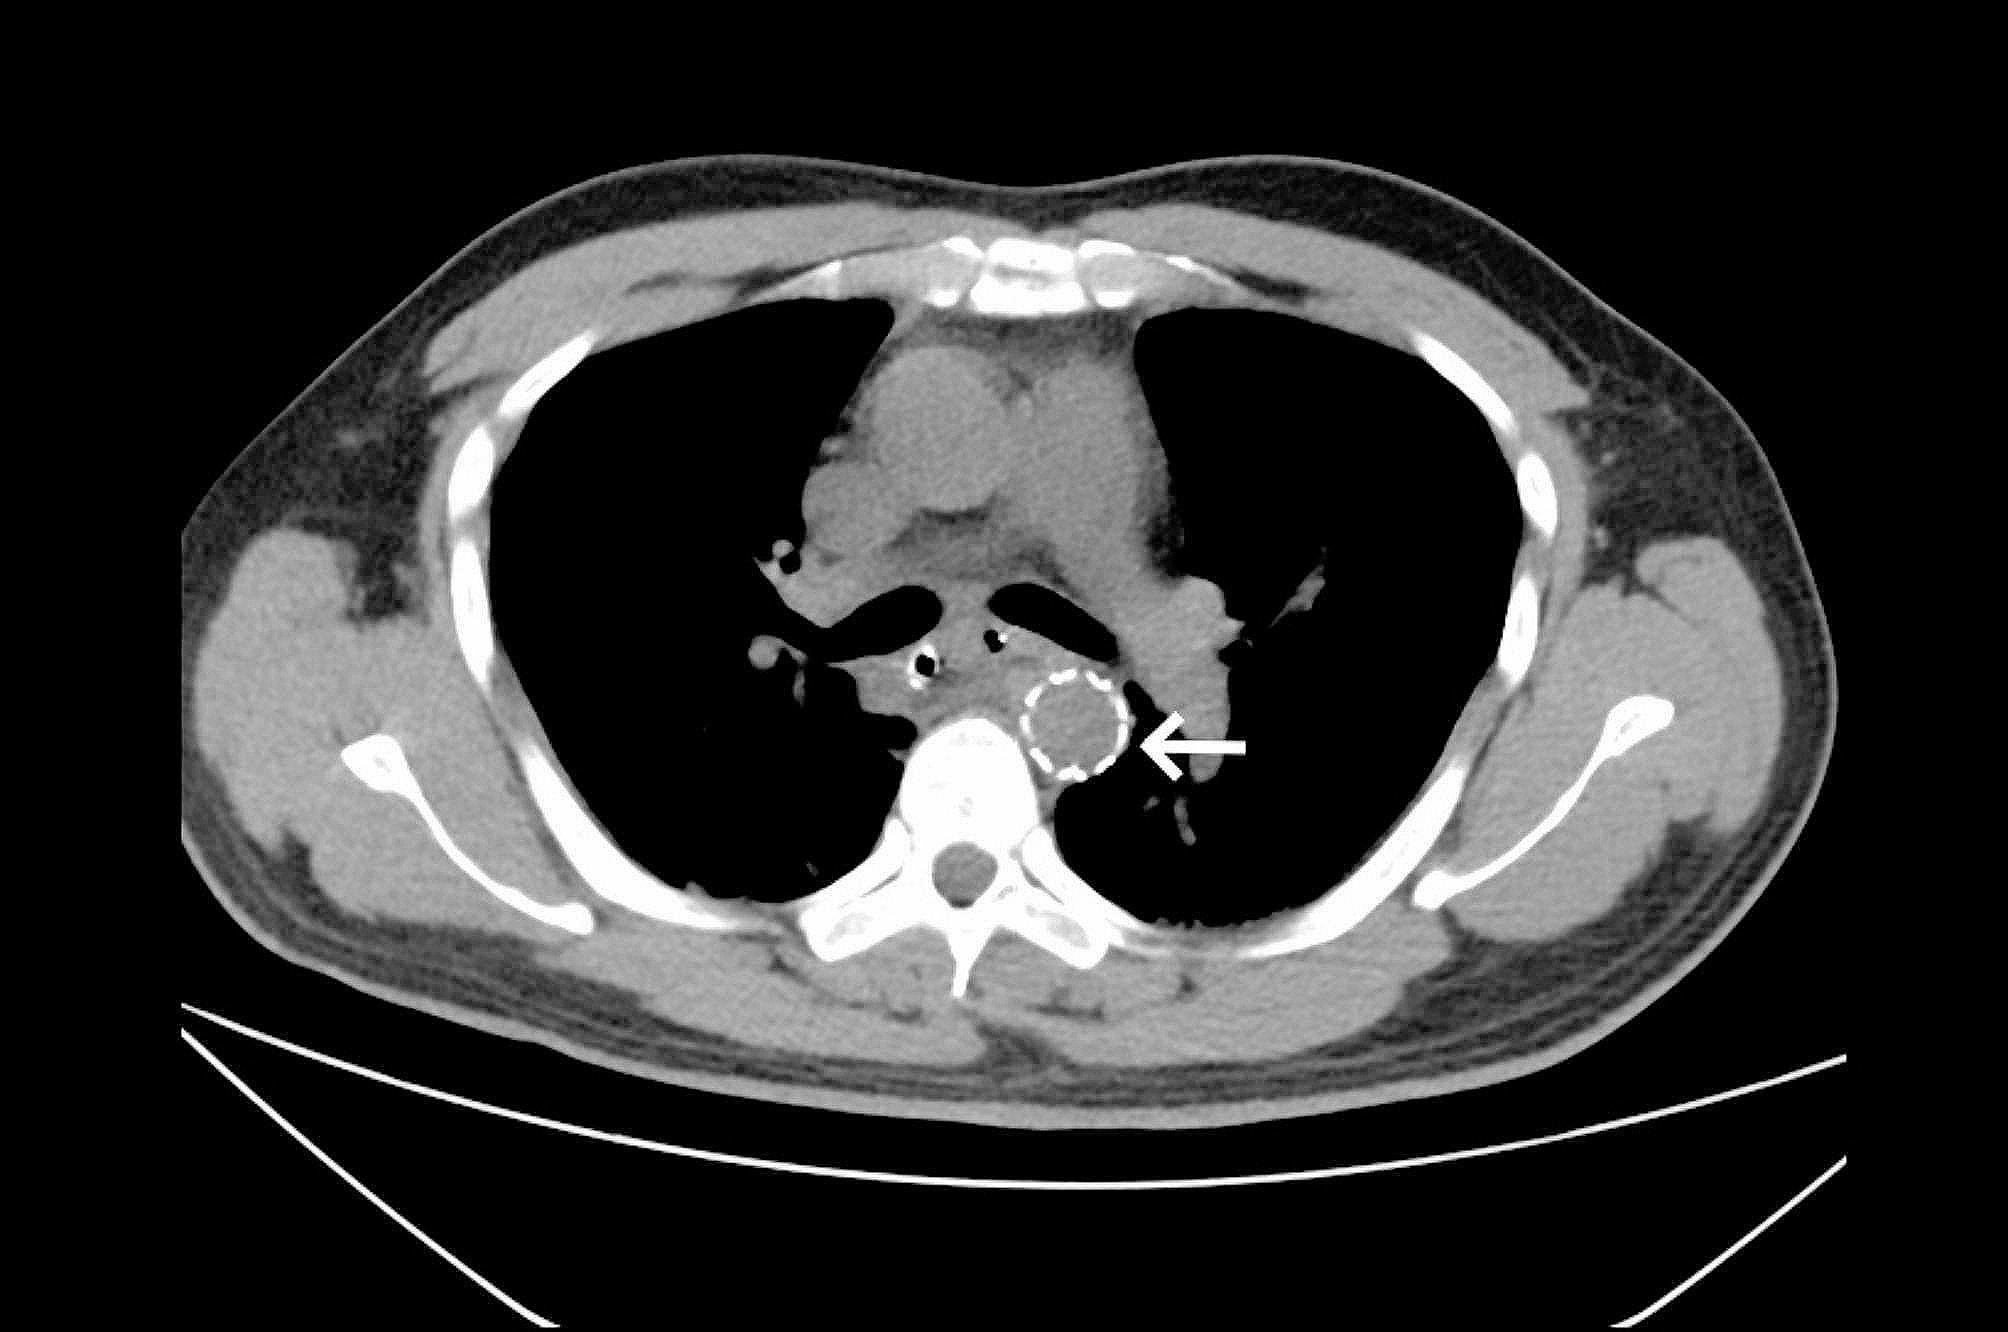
Computed tomography scan image. CT shows stent in descending aorta after surgery (white arrow)
